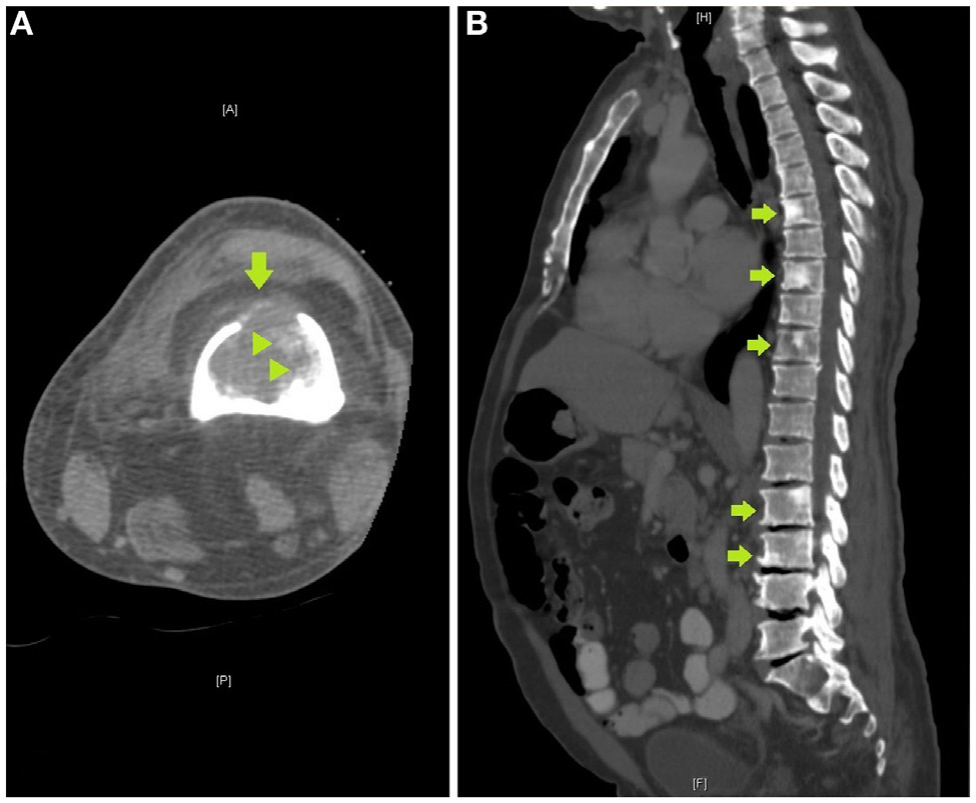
(A) Axial computed tomography (CT) image of the femur obtained to localize the lesion for CT-guided biopsy demonstrates a destructive lytic lesion with cortical breakthrough anteriorly (arrow) and areas of bone sclerosis (arrowheads), (B) sagittal image of a CT of the chest, abdomen, and pelvis demonstrates multiple sclerotic lesions in the spine, including within T6, T8, T10, L2, and L3 vertebrae (arrows).
